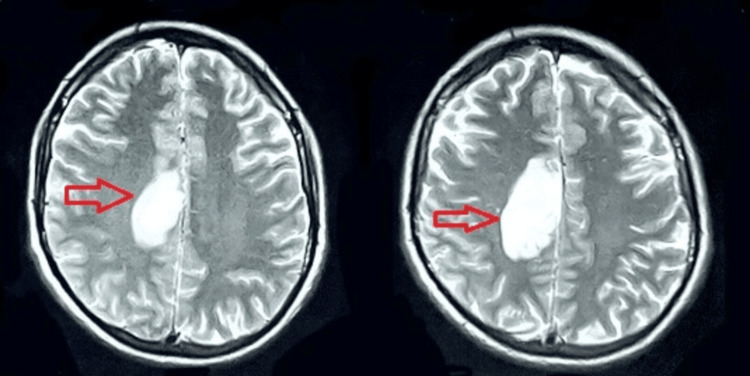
T2W MRI Brain Hyper-intense lesion in the frontoparietal region. T2WI: T2 weighted image
